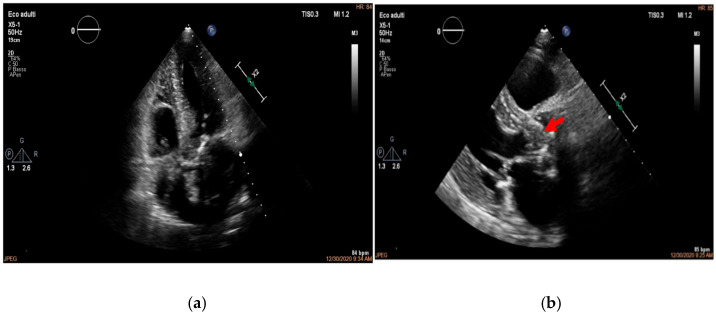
(a,b). Transthoracic echocardiogram showing aortic valve vegetation, more evident on the ventricular side of the aortic valve, as indicated by the arrow. This is an original, de-identified clinical image from our institution. Patient consent for publication was obtained.
